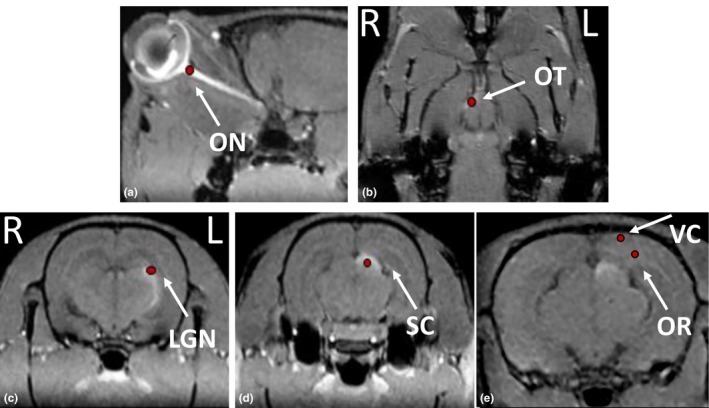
Representative manganese‐enhanced MR images to display the placement of regions of interest for the measurement of signal intensities. a, the level of optic nerve; b, the level of optic tract; c, the level of lateral geniculate nucleus; d, the level of superior colliculus; e, the level of optic radiation and visual cortex
